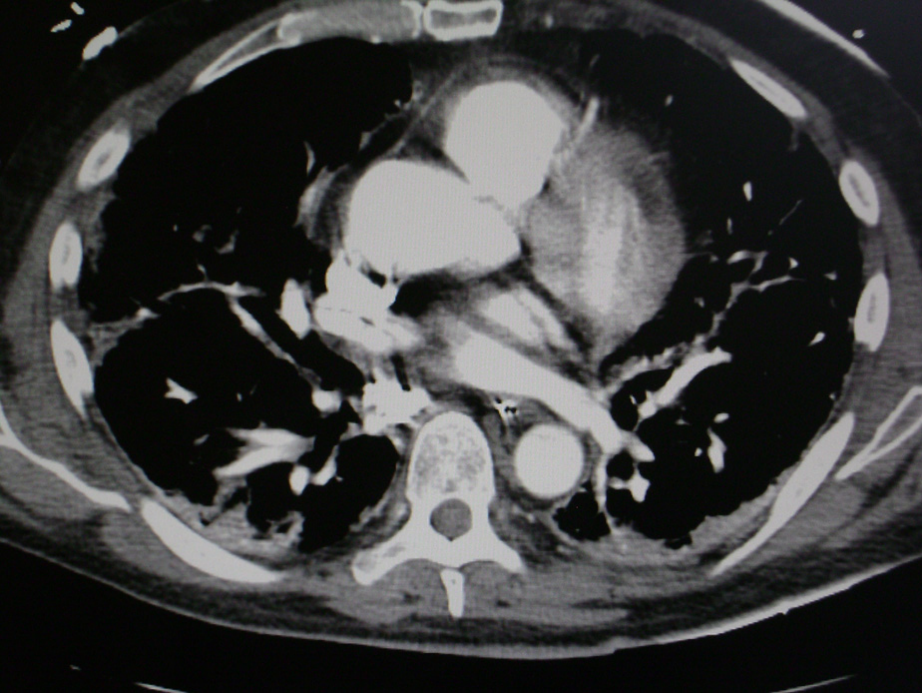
Interstitial pulmonary fibrosis in our patient's computed tomography scan of the chest.
